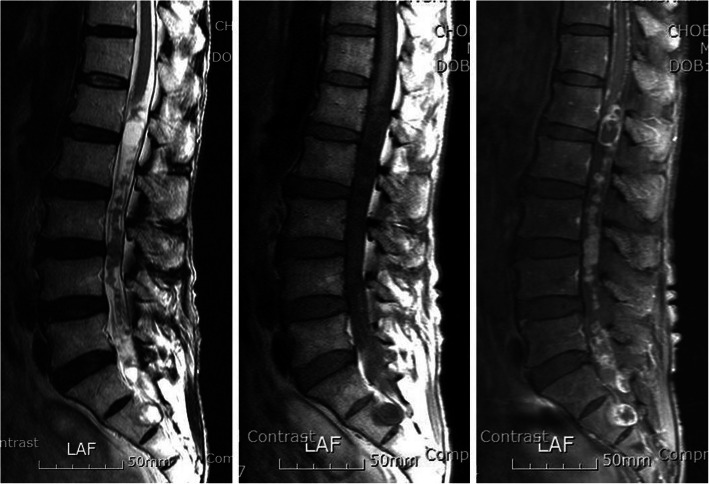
Preoperative magnetic resonance imaging shows large mass lesion with a continuous multi-lobulated bead-like mass and a cystic portion from L1 to S3. The lesion is iso-intense on T2-weighted images (WI), iso- to slightly low-intense on T1-WI, and heterogeneous enhancement on contrast-enhanced T1-WI
